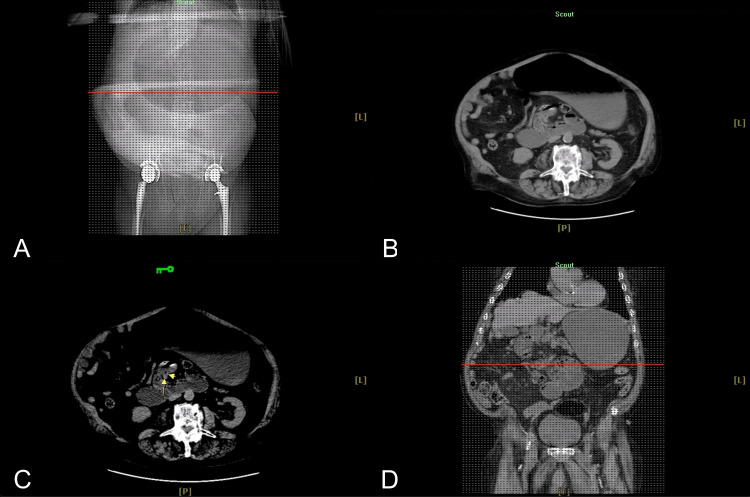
(A)-(D) Abdominopelvic CT scan without contrast shows midgut volvulus. Impressions: Fluid-filled distal esophagus and severely distended stomach with an air-fluid level. There is distended duodenum and proximal portion of the jejunum with the collapse of the rest of the small bowel and twisting of the superior mesenteric vessels. Small fat-containing ventral abdominal wall hernia in the suprapubic region. CT, computed tomography
